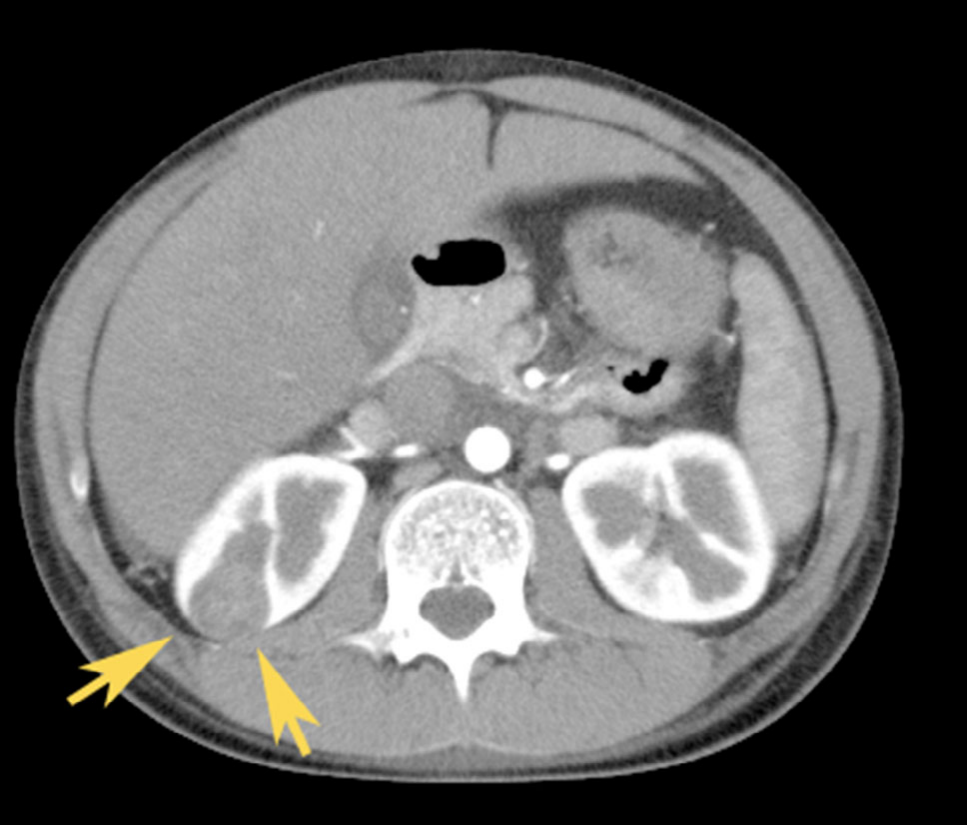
Enhanced CT showing a 2‐cm mass lesion with slight and uneven enhancement in right kidney. Arrows: tumor.
